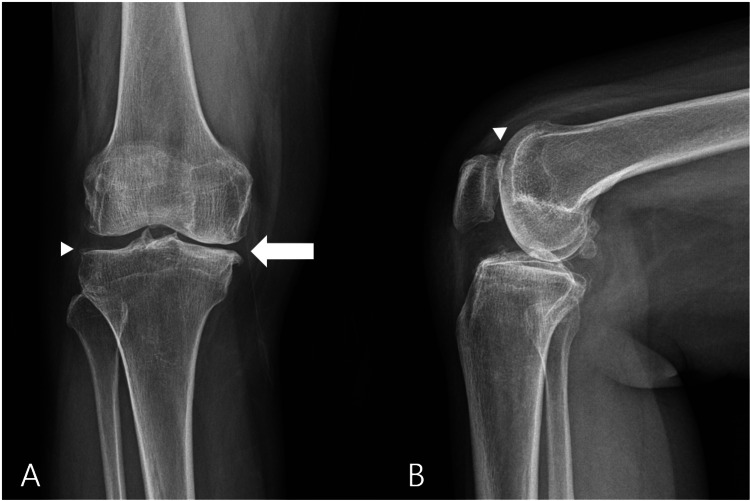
Follow-up radiographic findings of the right knee 22 months after treatment A: The anteroposterior radiograph demonstrates persistent medial compartment joint-space narrowing (white arrow) and marginal osteophyte formation (white arrowhead) without obvious interval progression compared with baseline imaging. B: The lateral radiograph demonstrates persistent degenerative osteoarthritic changes (white arrowhead) without obvious interval progression.
